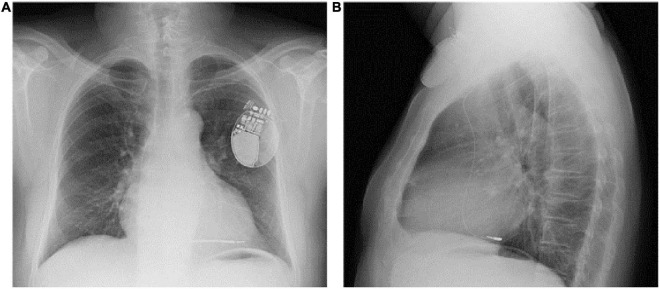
PA (A) and LL (B) chest x-ray of the patient showing inadvertent lead placement in the posterior branch of the coronary sinus. PA, posteroanterior; LL, laterolateral.
